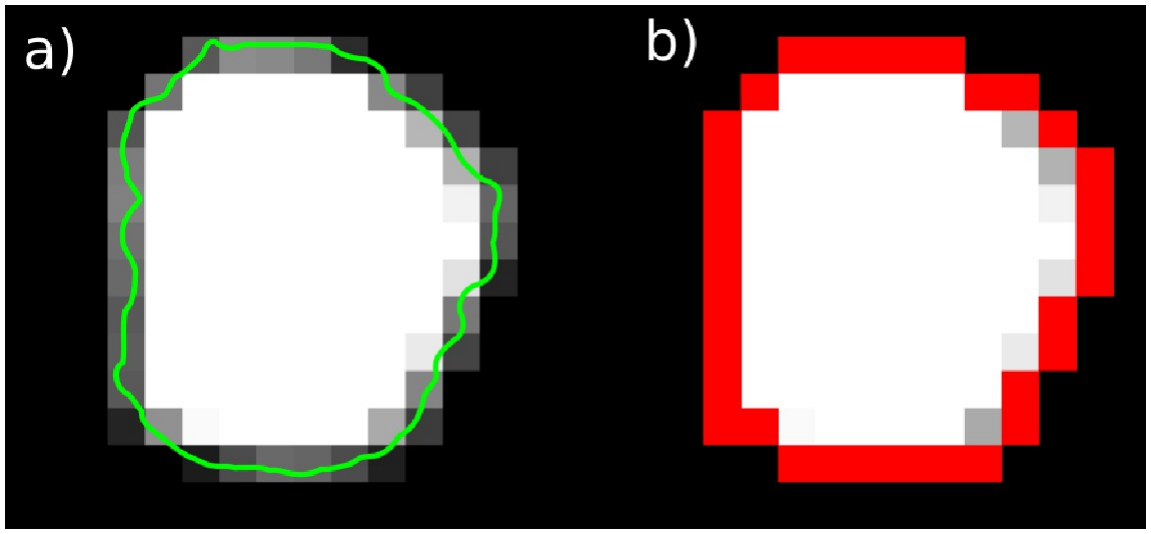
Surface partial volume effect. When imaging a real-world object (green outline) with a limited spatial resolution, pixels on the surface contain a mixture of object and the surrounding (“partial volume effect”). This effect is further enhanced by additional blurring inherent to CT scans. We introduced an approach where the objects are segmented by first using a relatively low HU threshold, and subtracting surface pixels (red) multiplied with a global factor, see text.
